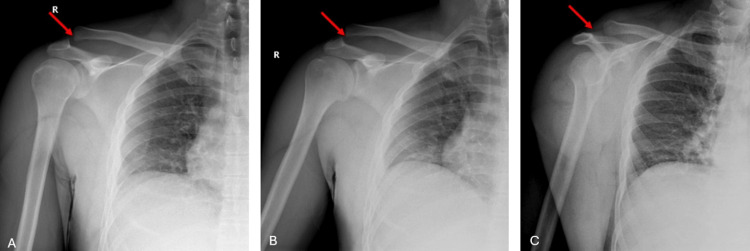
Initial shoulder radiographs of the patient (A) AP view. (B) True AP view. (C) Axial rotation view. All images demonstrate an AC joint dislocation. The red arrows indicate the AC joint separation. AC, acromioclavicular
